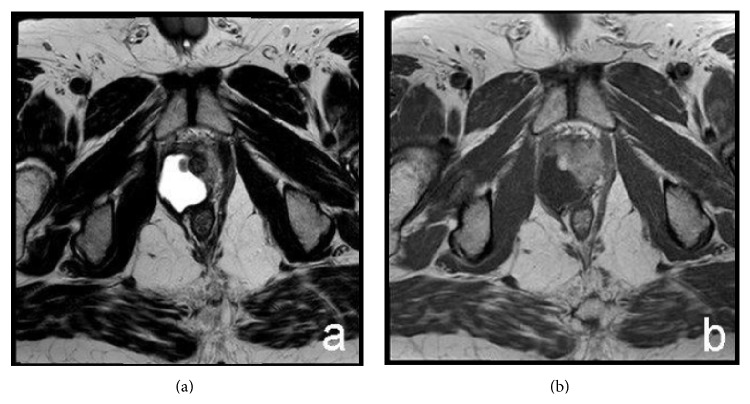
MRI. Postoperative images. Axial T2-weighted (a) and T1-weighted after contrast administration (b) images. Persistence of synovial sarcoma in the prostatic loggia.
